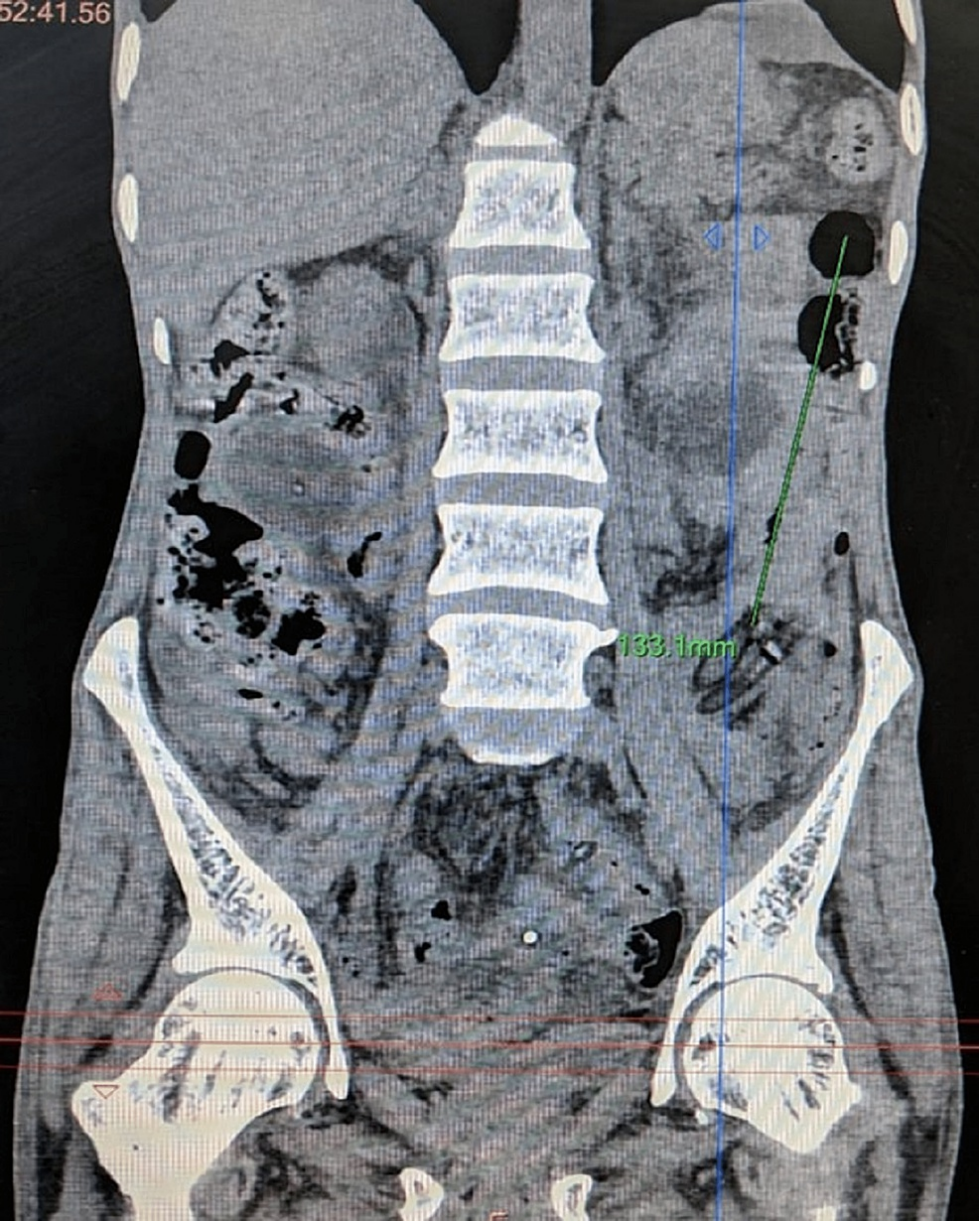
Contrast-enhanced CT (coronal section) showing a displacement of 133.1 mm of Foley catheter from the jejunotomy site.
